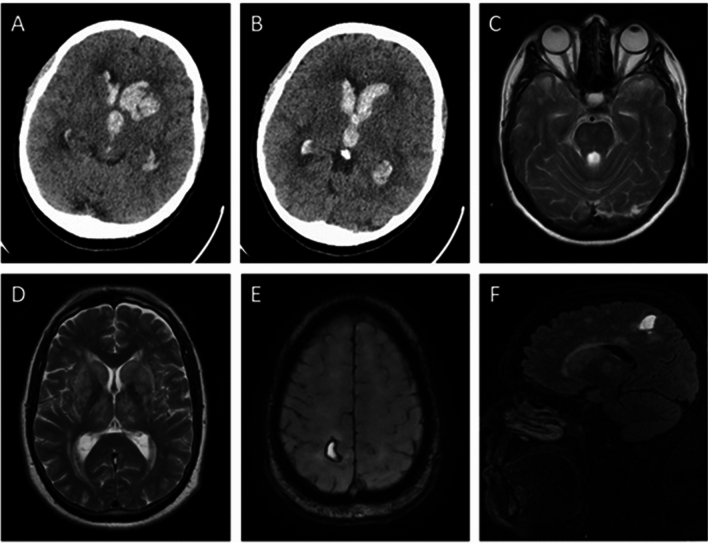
A–C Brain imaging findings of patient A1. A non-contrast CT scan A, B revealed a left caudate haemorrhage with intraventricular extension. T2-weighted MRI sequences C demonstrated diffuse WMH with bilateral temporal pole involvement. D–F Brain imaging findings of patient A4. T2-weighted MRI D demonstrated diffuse WMH involving bilateral external capsules. Susceptibility-weighted E and T1-weighted F MRI sequences revealed ICH at the subcortical white matter of right superior parietal lobule
